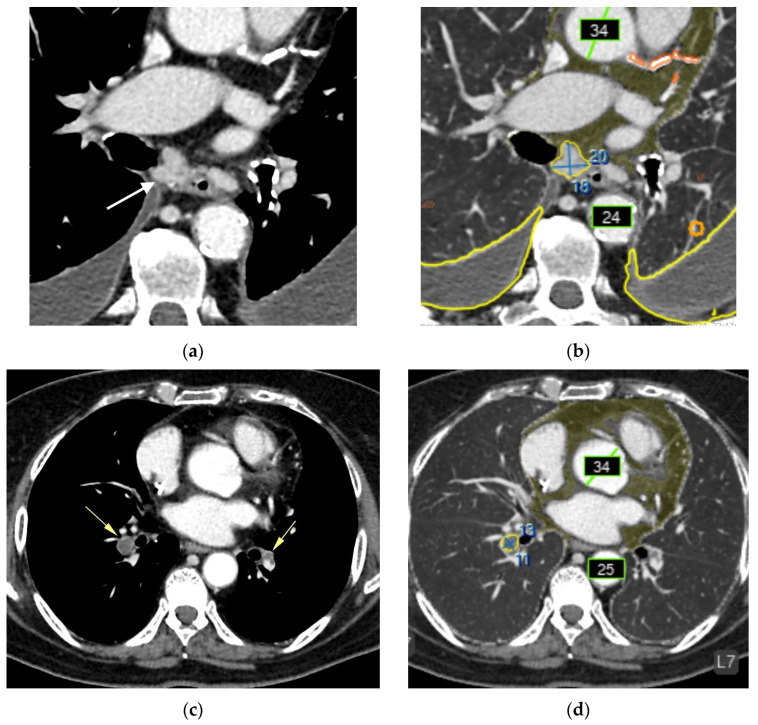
Chest CT before (a,c) and after AI processing (b,d). Intermediate errors. (a,c) Two adjacent, non-enlarged bifurcation lymph nodes (white arrow) are segmented by the AI as a single enlarged node (false-positive error). Although a radiologist can recognize this error, it may be challenging for less experienced readers. The AI service correctly identified bilateral pleural effusion (blue contour), measured the diameters of the ascending and descending thoracic aorta (green), coronary calcifications (orange), paracardial fat (dark green), emphysema (brown), and a small focus of pulmonary infiltration associated with COVID-19. (b,d) A thrombus in the right pulmonary artery (yellow arrow) is misclassified as enlarged intrathoracic lymph nodes (false-positive error). The AI was not trained to detect pulmonary artery thrombi but was trained to identify enlarged lymph nodes in this region. Due to limited training examples containing both lymphadenopathy and thrombi, the model misinterpreted the lesion as lymph node enlargement. Nevertheless, the detected abnormality could prompt a radiologist to recognize a potential thrombus. The AI correctly measured the diameters of the ascending and descending thoracic aorta (green), coronary calcifications (orange), and paracardial fat (dark green).
